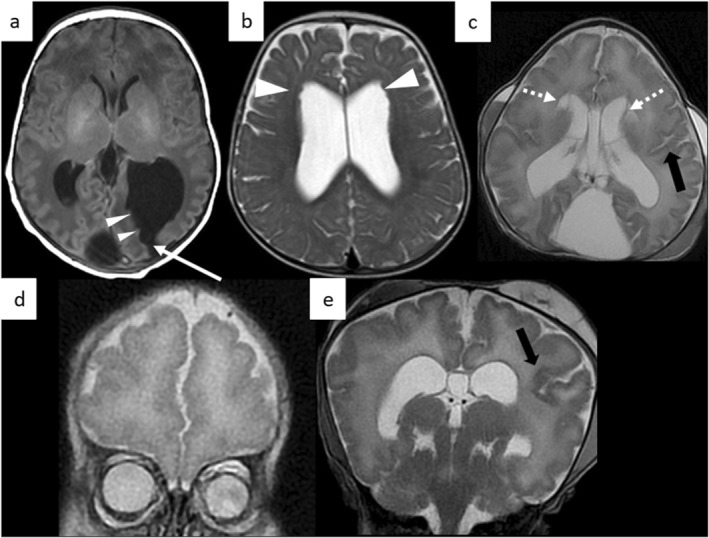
Postnatal MRI showing associated cerebral malformations. (a) Patient L13 (BPC). T1‐weighted axial image showing left occipital schizencephaly (white arrow) and subependymal nodular heterotopia (arrowheads) along the enlarged left occipital horn. (b) Patient TRS13 (unclassified DWM or BPC). T2‐weighted axial slice showing enlarged lateral ventricles with bilateral frontal subependymal nodular heterotopia (arrowheads). (c–e) Patient TRS14 (DWM). T2‐weighted axial (c) and coronal (d, e) planes showing bilateral frontal periventricular pseudocysts (dotted arrows) and left perisylvian polymicrogyria (black arrow) (right perisylvian polymicrogyria is not clearly visible on these slices). The olfactory bulbs and sulci were not visible in this patient.
